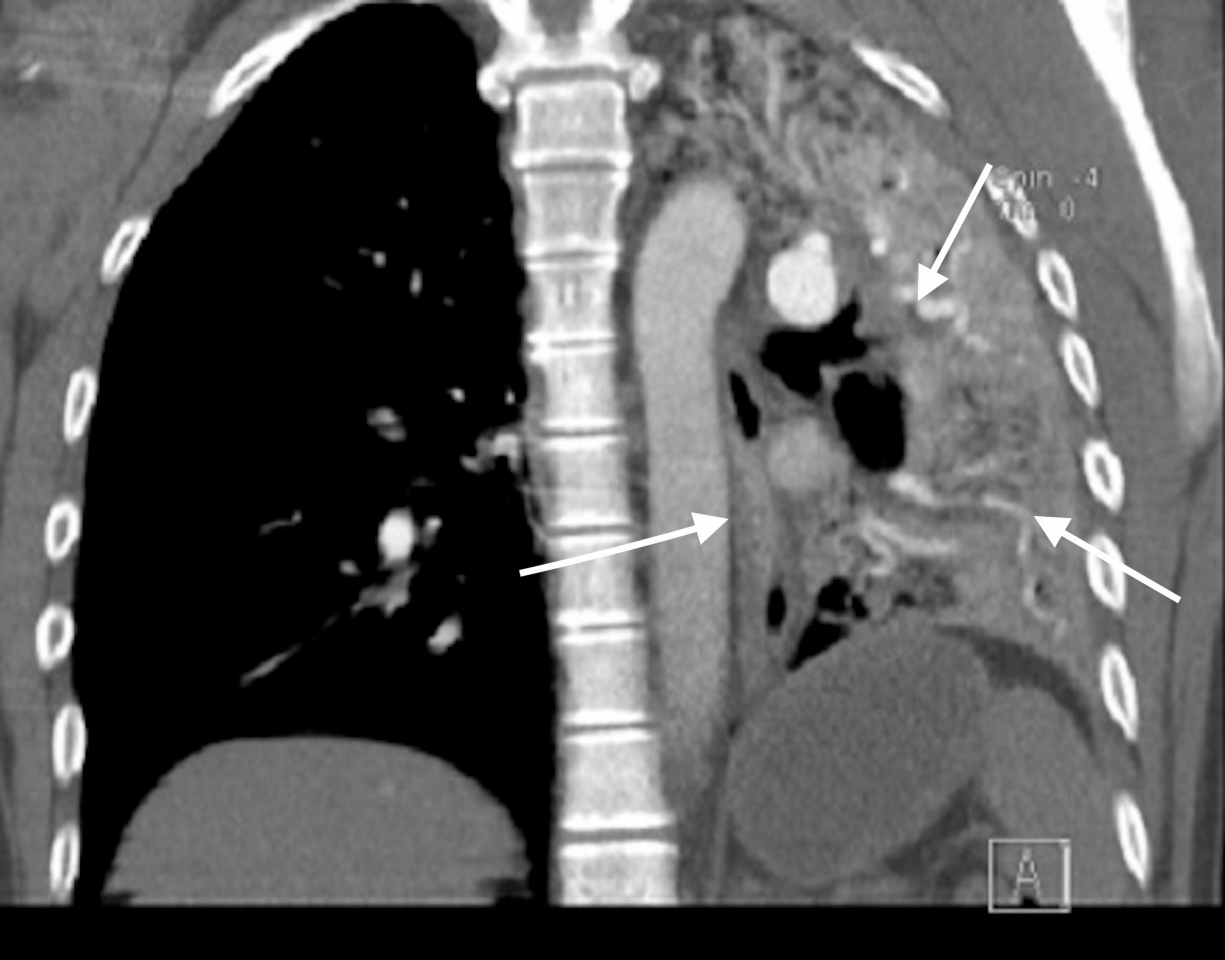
Chest CT (coronal view) showing reduced volume of the left lung with near-total involvement by confluent consolidations extending to the pulmonary periphery, consistent with diffuse alveolar hemorrhage. No intraluminal filling defects are observed that would suggest pulmonary embolism (PE). There is a decrease in volume of the left lung with almost total involvement by confluent consolidations extending to the pulmonary periphery, compatible, within an appropriate clinical context, with alveolar hemorrhage associated with drug use. The tracheobronchial tree is patent up to its tracheal bifurcation. No supraclavicular, axillary, hilar, or mediastinal lymphadenopathy of significance by size criteria is observed. The heart and great vessels show no abnormalities. There is no pericardial effusion.
